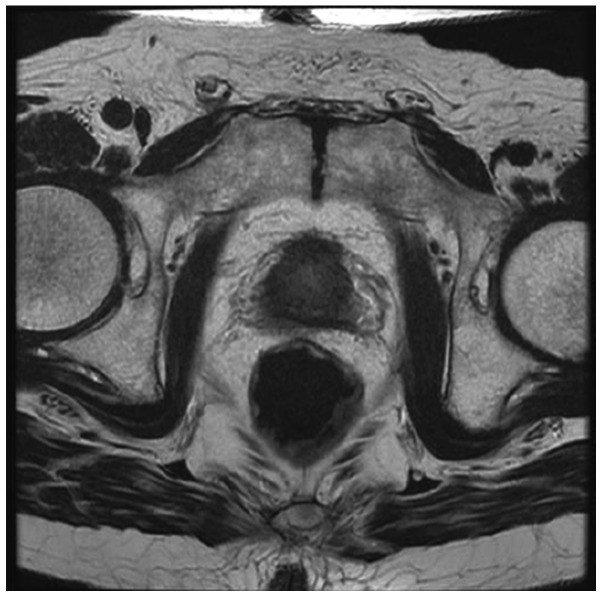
Pelvic magnetic resonance imaging following 8 months of androgen deprivation therapy showing shrinkage of the cyst.
